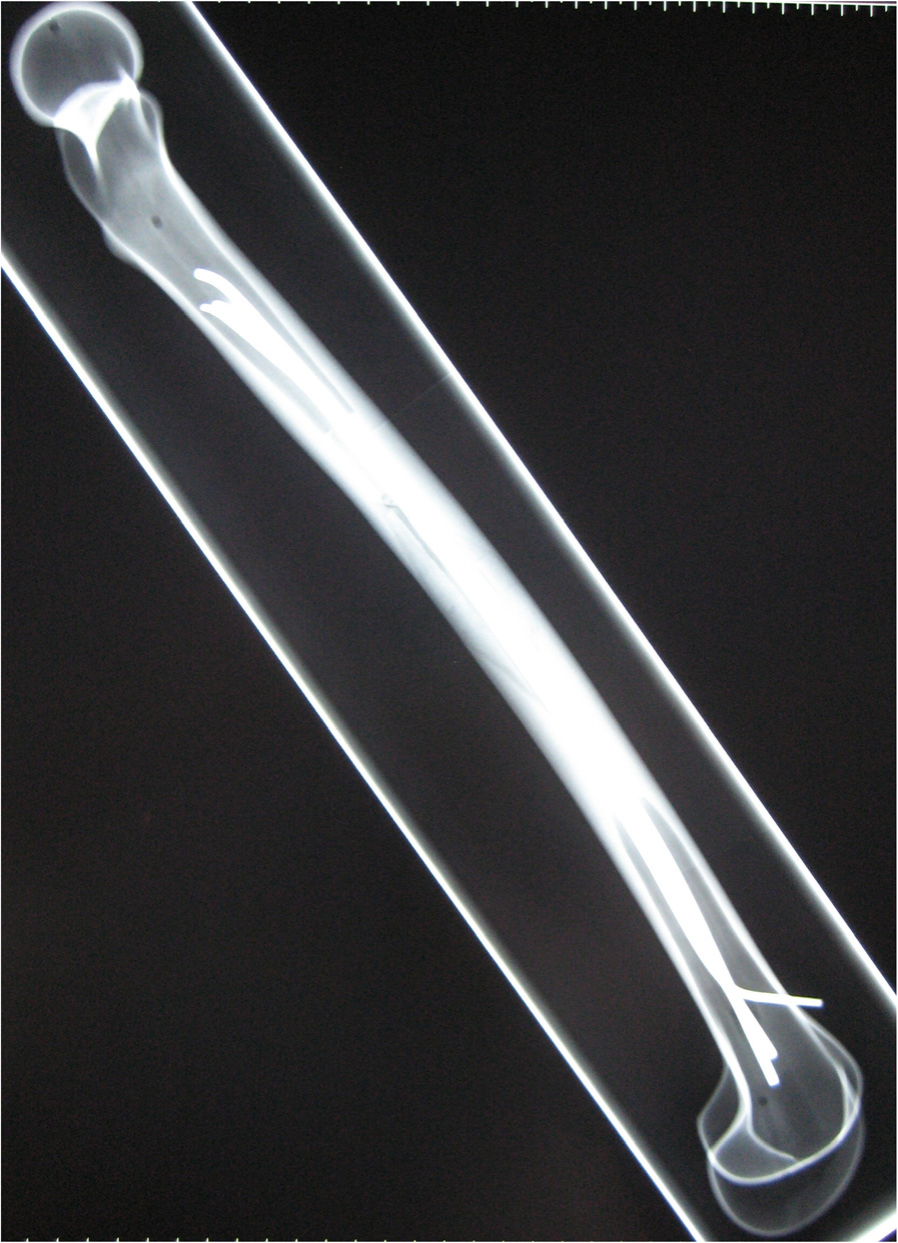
Radiograph (lateral view) of a 3CL femoral model with retrograde intramedullary nailing, showing two 3.5 mm titanium nails implanted in 2C shaped configuration and one additional 2.5 mm titanium nail implanted antero-lateral.
